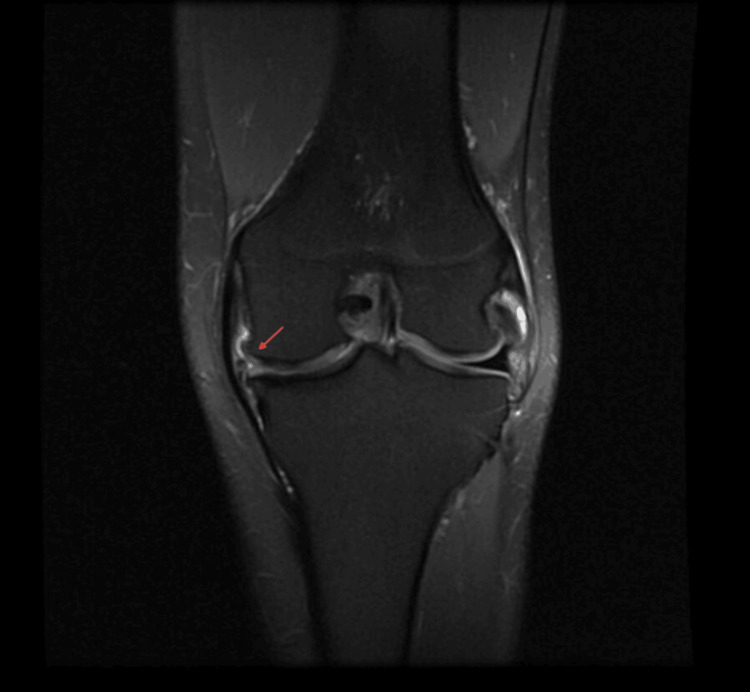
Magnetic resonance imaging status post graft resection Subtotal meniscectomy of the medial meniscus (red arrow). Coronal view of the left knee.
